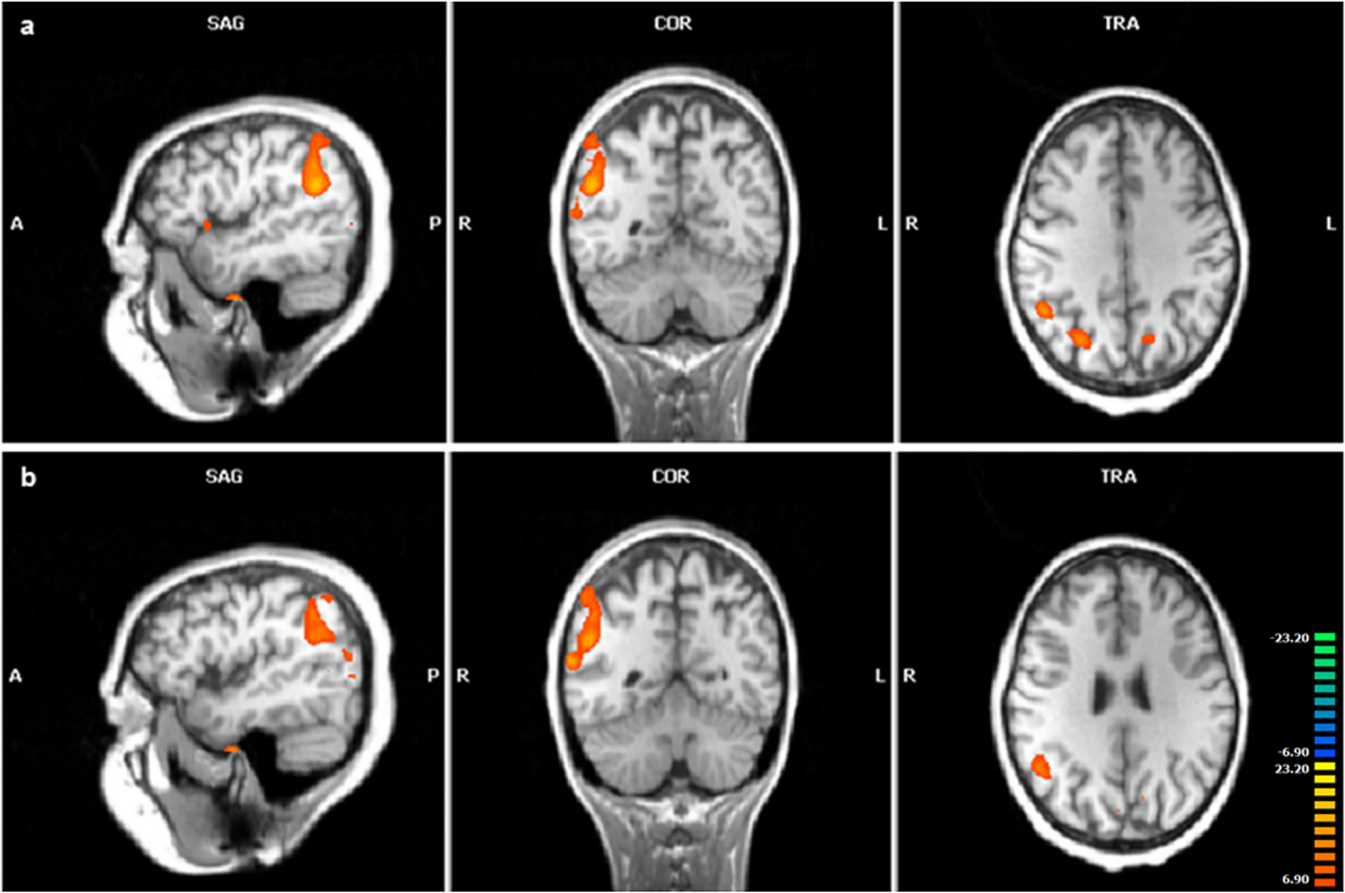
Functional MRI. Functional MRI results during a working memory task (2- minus 0-back) are demonstrated for sagittal (SAG), coronal (COR) and transversal (TRA) sections. (a: anterior; p: posterior; r: right; l: left). ANCOVA was calculated including haplotype status, age, sex and education as covariates (*T*-score: 6.9–23.2; *p*_(uncor)_ <0.01). a Haploblock 2: GAGTAG carriers were analyzed vs. non-carriers (talairach coordinates: *x* = 44; *y* = −53; *z* = 34). b Haploblock 3: CAACCGGG carriers were analyzed vs. non-carriers (talairach coordinates: *x* = 44; *y* = −50; *z* = 28).
